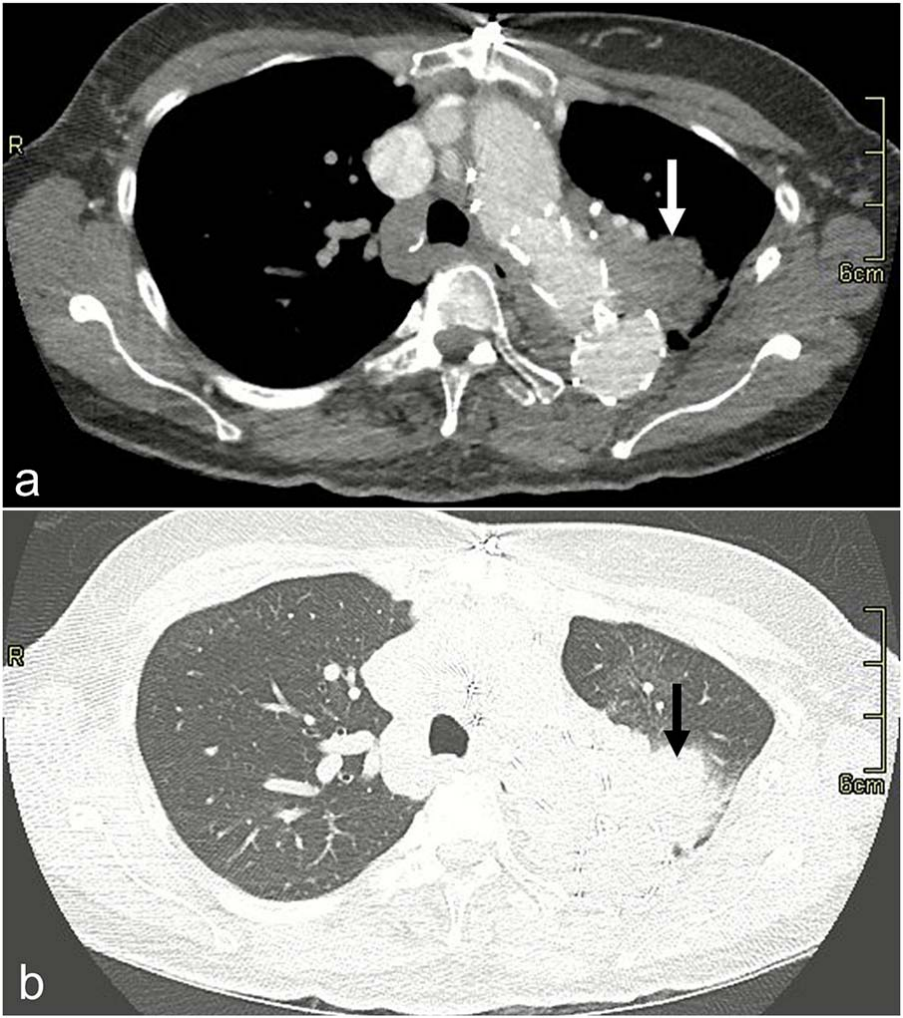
CT scan image with dissection protocol of the left upper lobe consolidation (arrow) on (a) mediastinal and (b) lung windows.
